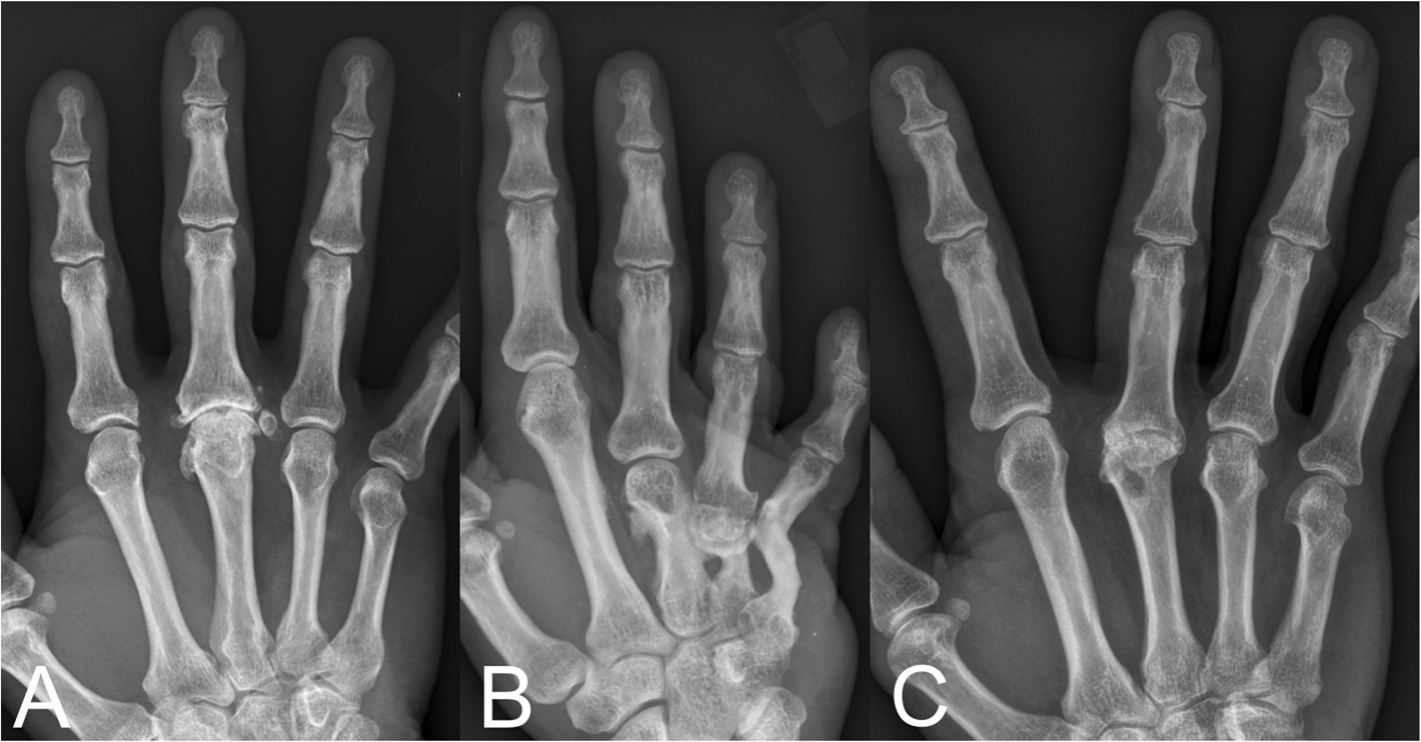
Radiographs of the reconstructed metacarpophalangeal joints in case 9–11 (a-c). The joint space in case 10 (MCP IV) was difficult to visualize due to the bony deformities caused by the shotgun injury in 1978
